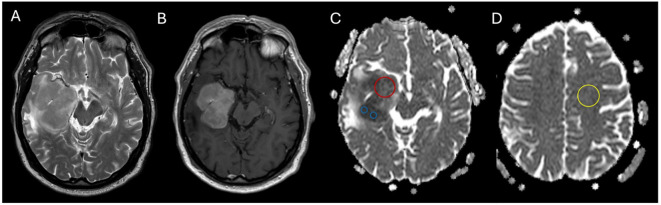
Region-of-interest (ROI) placement for apparent diffusion coefficient (ADC) analysis. Axial T2-weighted (A) and post-contrast T1-weighted (B) images show an enhancing tumour in the right temporal lobe. ADC maps (C, D) demonstrate ROI placement: a large red ROI for ADCmean and small blue ROIs for ADCmin (C), and a yellow ROI in the contralateral normal-appearing white matter at the level of the centrum semiovale (D). Up to three ADCmin ROIs were placed in areas of greatest diffusion restriction, which were not necessarily within the same slice.
